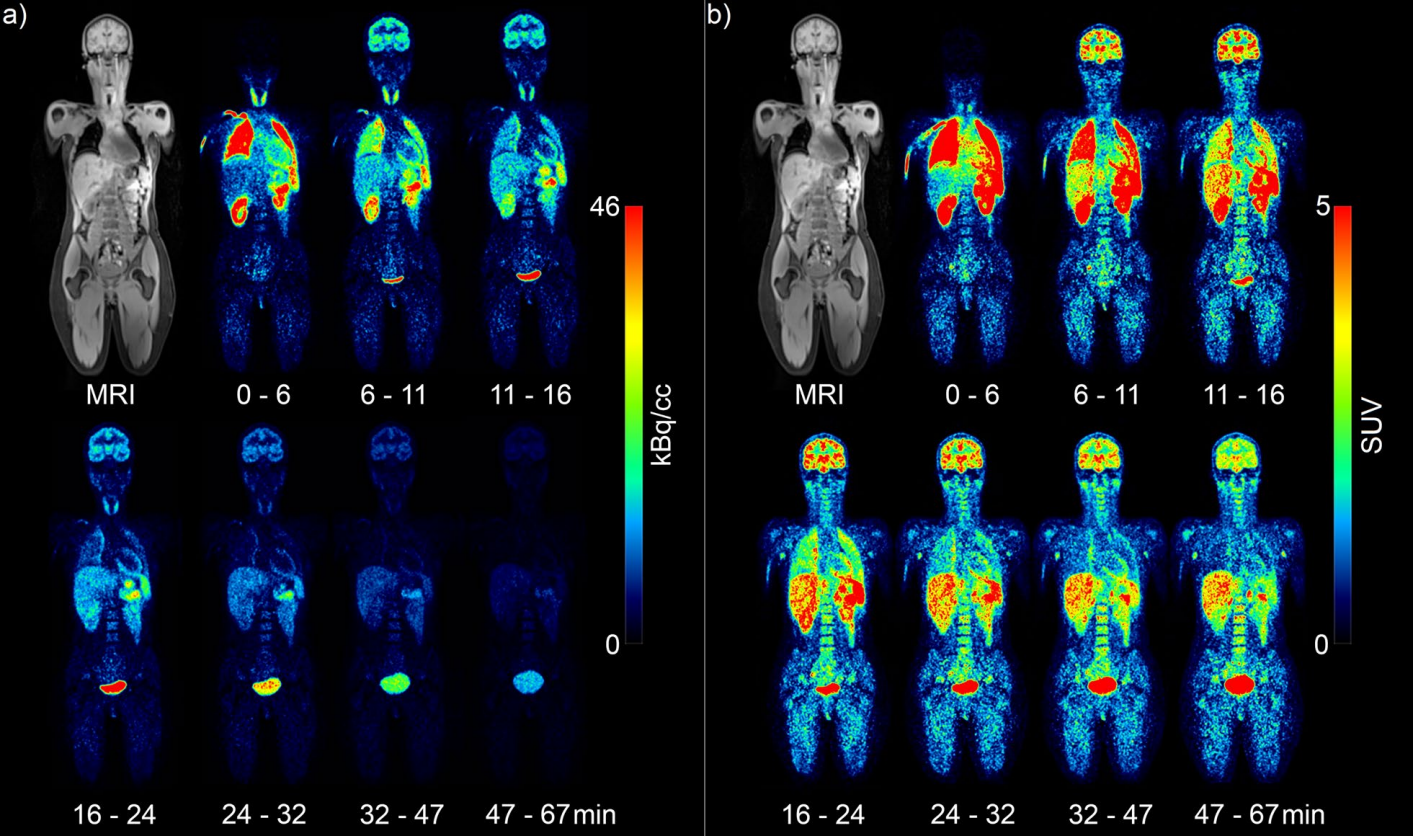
Tracer distribution over time of one representative subject. Depicted is an MR image for anatomical comparison to the PET images: a activity in kBq/cc (not decay corrected); b standardized uptake value (SUV; decay corrected). The radioligand *(R)*-[^11^C]Me-NB1 showed high initial uptake in the thyroid, lungs, spleen, pancreas and kidneys. In contrast, activity in the bone marrow (substituted by the vertebrae L4 and L5) and the liver built up over a longer period. Increase in the bladder volume over time is clearly visible. The first seven cycles are shown because of the advanced time and the low activity in the last cycles
